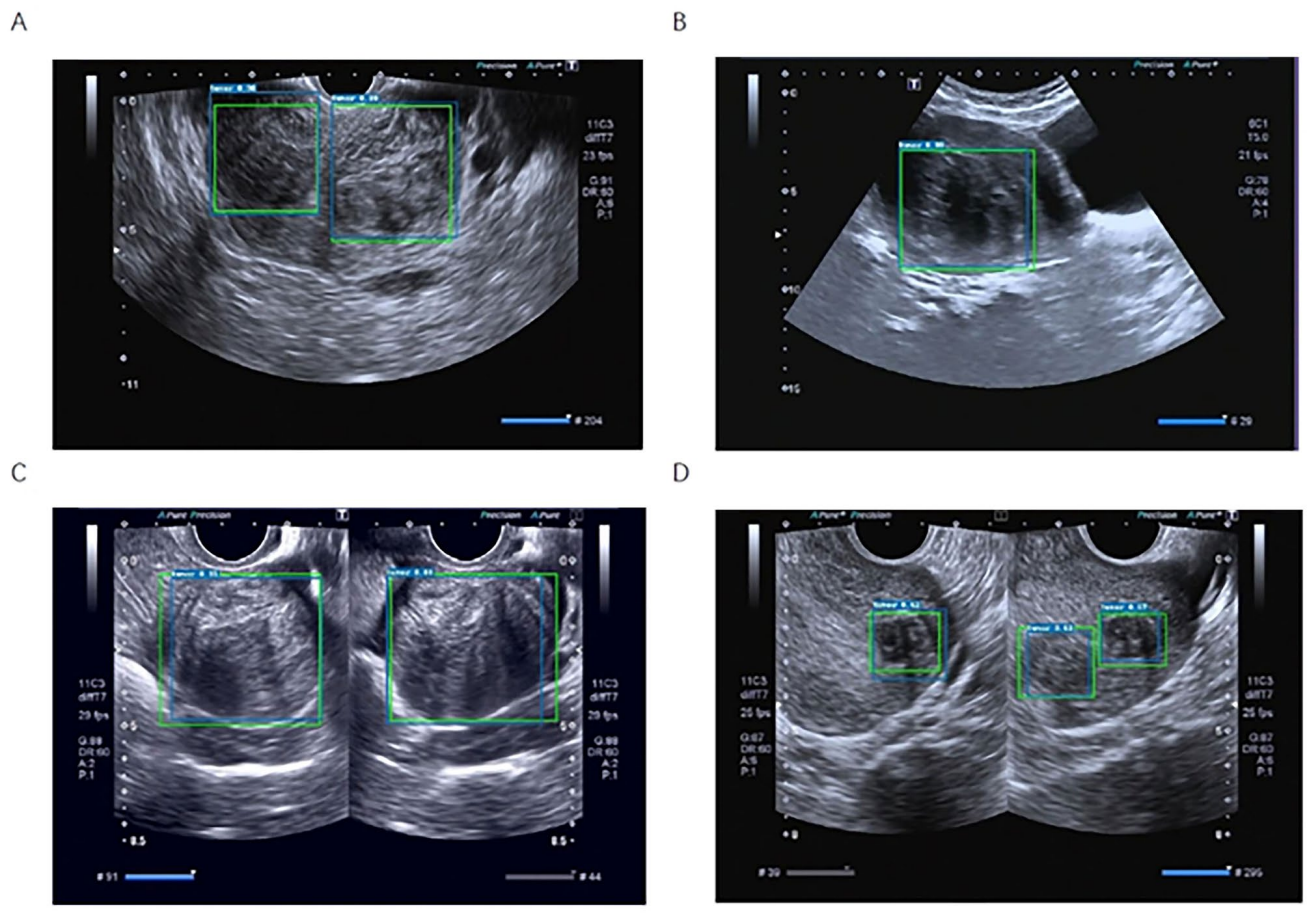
Uterine fibroid detection results. (A) Target detection results of multiple uterine fibroids in a single ultrasound image. (B) Target detection results of a single uterine fibroid in a single ultrasound image. (C) Target detection results of single uterine fibroids in patients with double ultrasound images. (D) Target detection results of multiple uterine fibroids in patients with double ultrasound images. Green boxes are the GT boxes annotated by our invited radiologists, and blue boxes are the boxes predicted by the trained model. The number marked in the upper left corner is the model detection confidence score.
